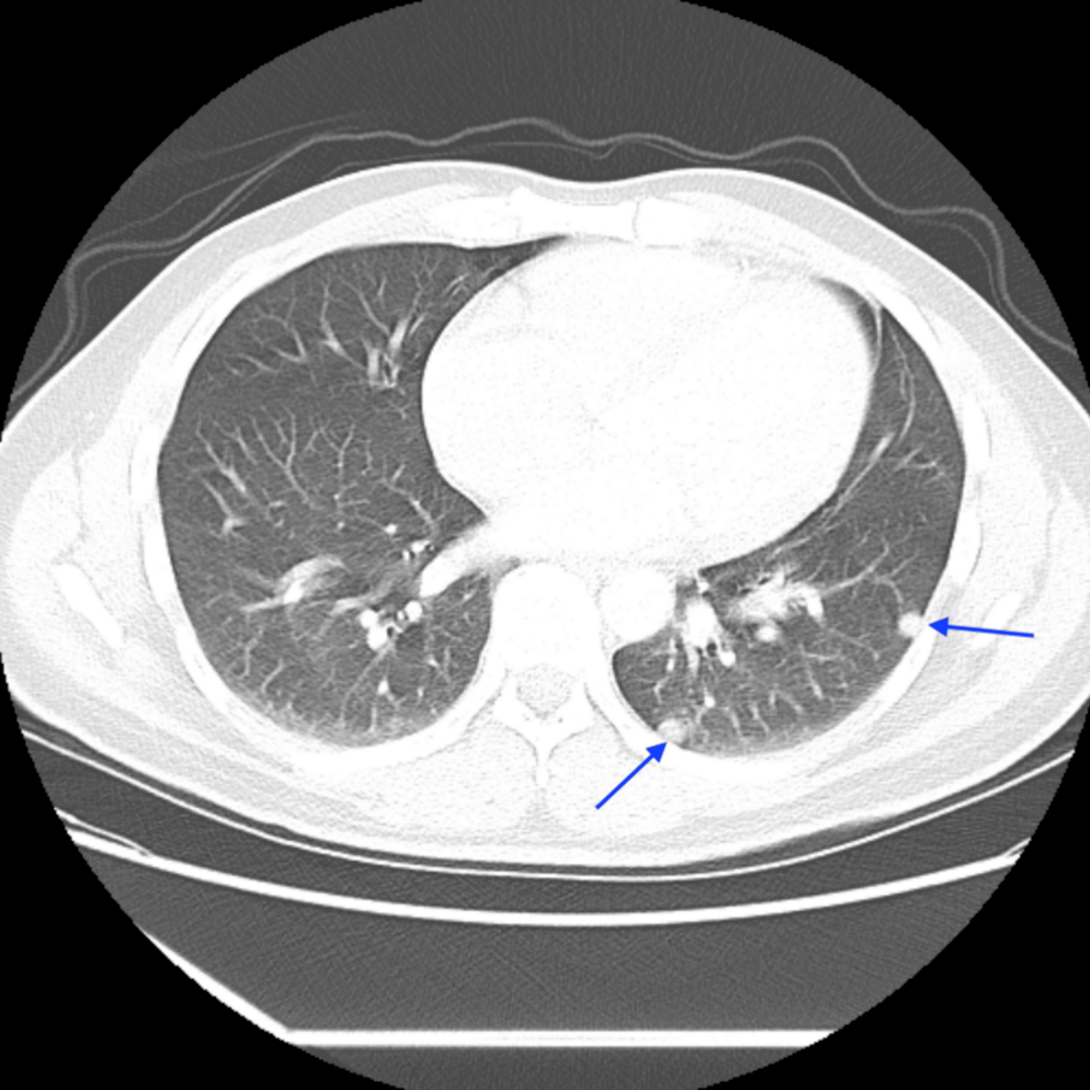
Computed tomography (CT) scan of the chest showing metastases (blue arrows)
